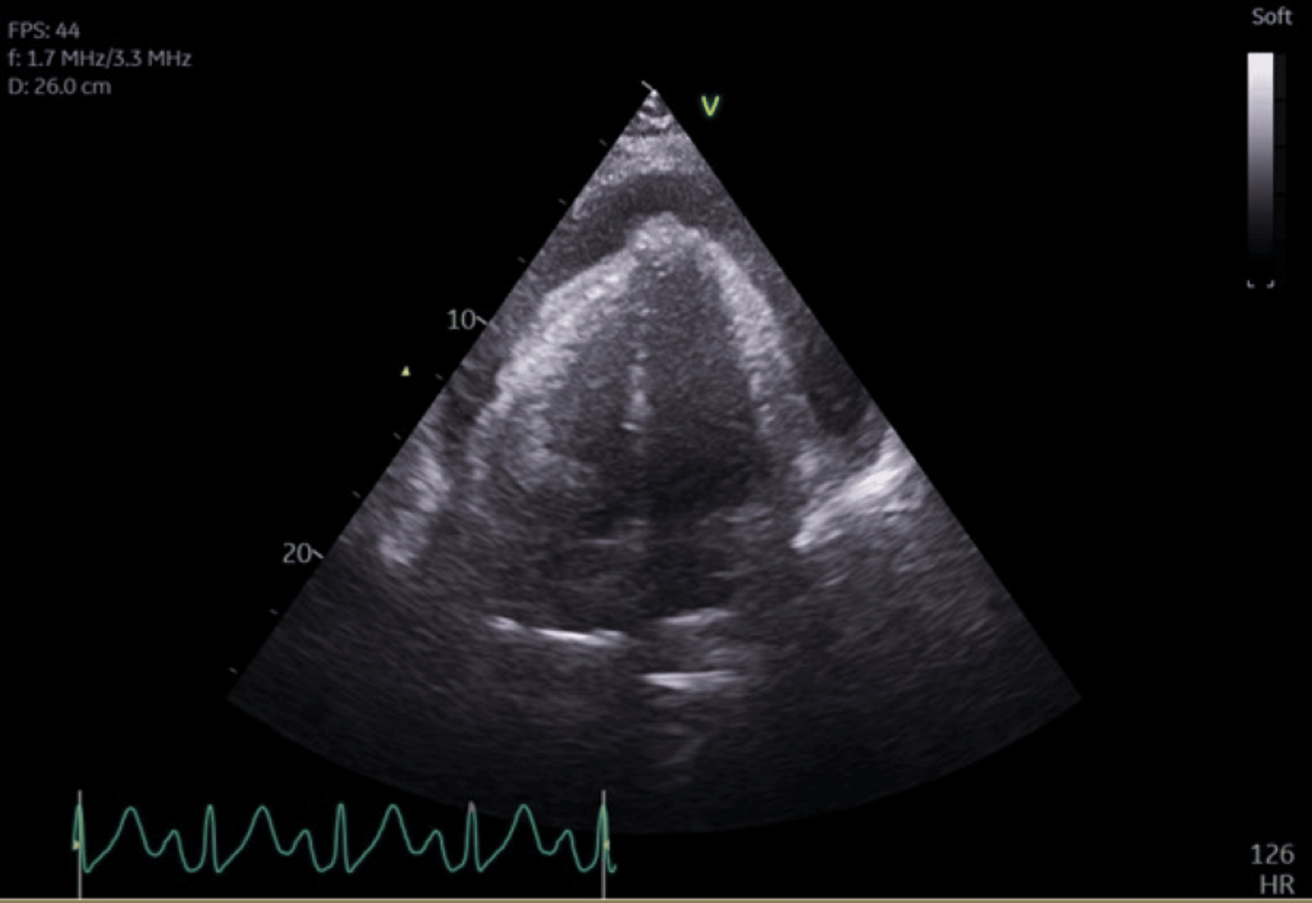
Apical four-chamber view showing a large global pericardial effusion FPS: frames per second, V: velocity, HR: heart rate, f: frequency, D: depth.
